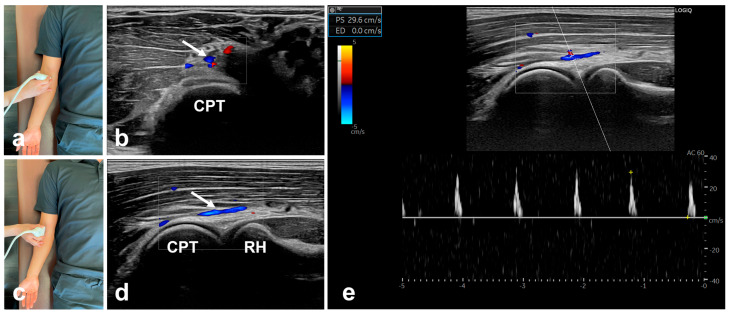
Peak systolic velocity (PSV) measurements of the radial recurrent artery (RRA). (a) Transducer position for short-axis view of the RRA; (b) Short-axis view of the RRA; the white arrow shows the RRA; (c) Transducer position for longitudinal-axis view of the RRA; (d) Longitudinal axis-view of the RRA; the white arrow shows the RRA; (e) Pulse Doppler sonograms of the RRA. PSV, peak systolic velocity; RRA, radial recurrent artery. CPT, capitellum of the humerus; RH, radial head.
